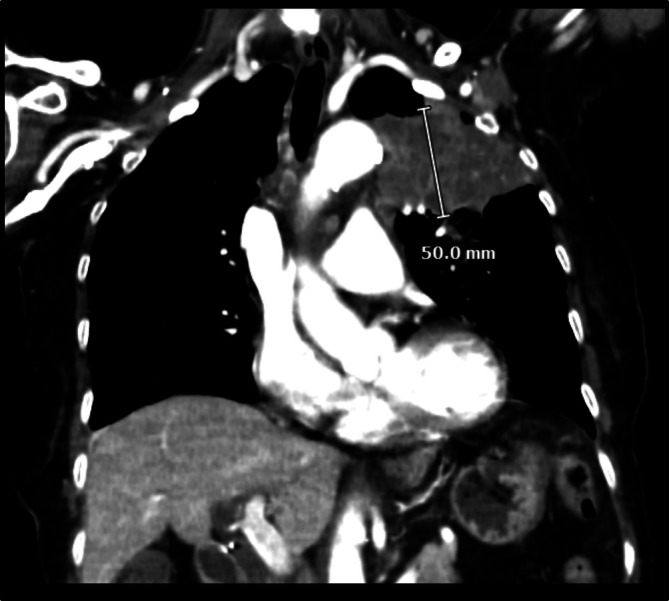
CT scan of the chest with contrast indicating a soft tissue density in the left upper lobe.
